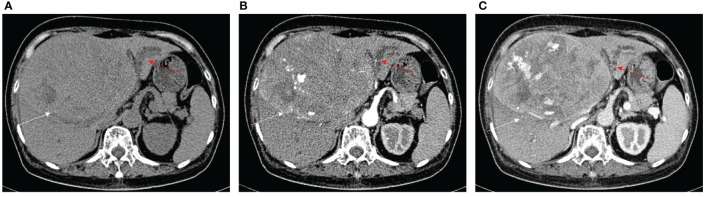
HCC without BDTT. (A) The HCC lesion (white arrow) and bile duct dilation in S2, S3, and S4 (red arrow) show hypoattenuation in plain CT image. (B) The HCC lesion has early enhancement (white arrow) in arterial phase, and bile duct dilation in S2, S3, and S4 (red arrow). (C) The HCC lesion (white arrow) shows hypoattenuation at portal venous phase, accompanied by closed bile duct dilation (red arrow) in S2, S3, and S4.
